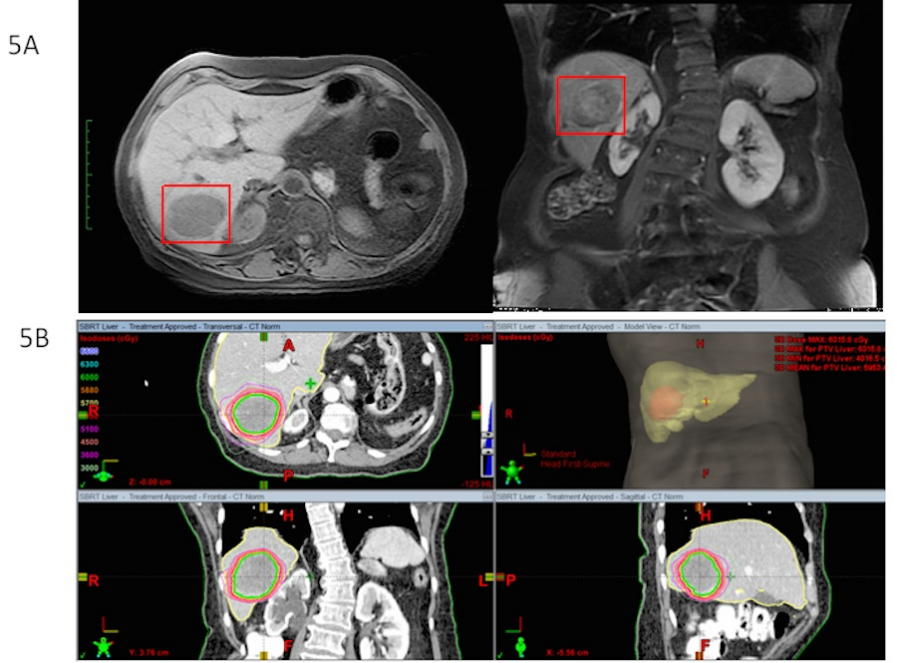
Liver MRI and SBRT Treatment Planning 5A) Large lesion in the right lobe of the liver (segment 7/6). 5B) Treatment planning SBRT for liver metastasis. Dose: 20 Gy x 3 fractions. Total dose: 60 Gy.
